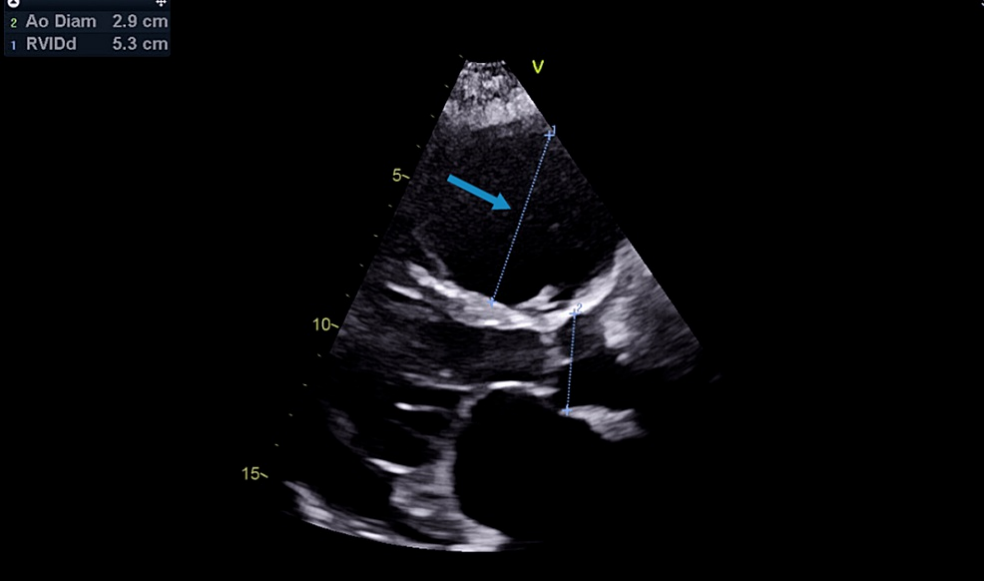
Echocardiogram (image 2) The blue arrow shows dilated right ventricle (RV).
